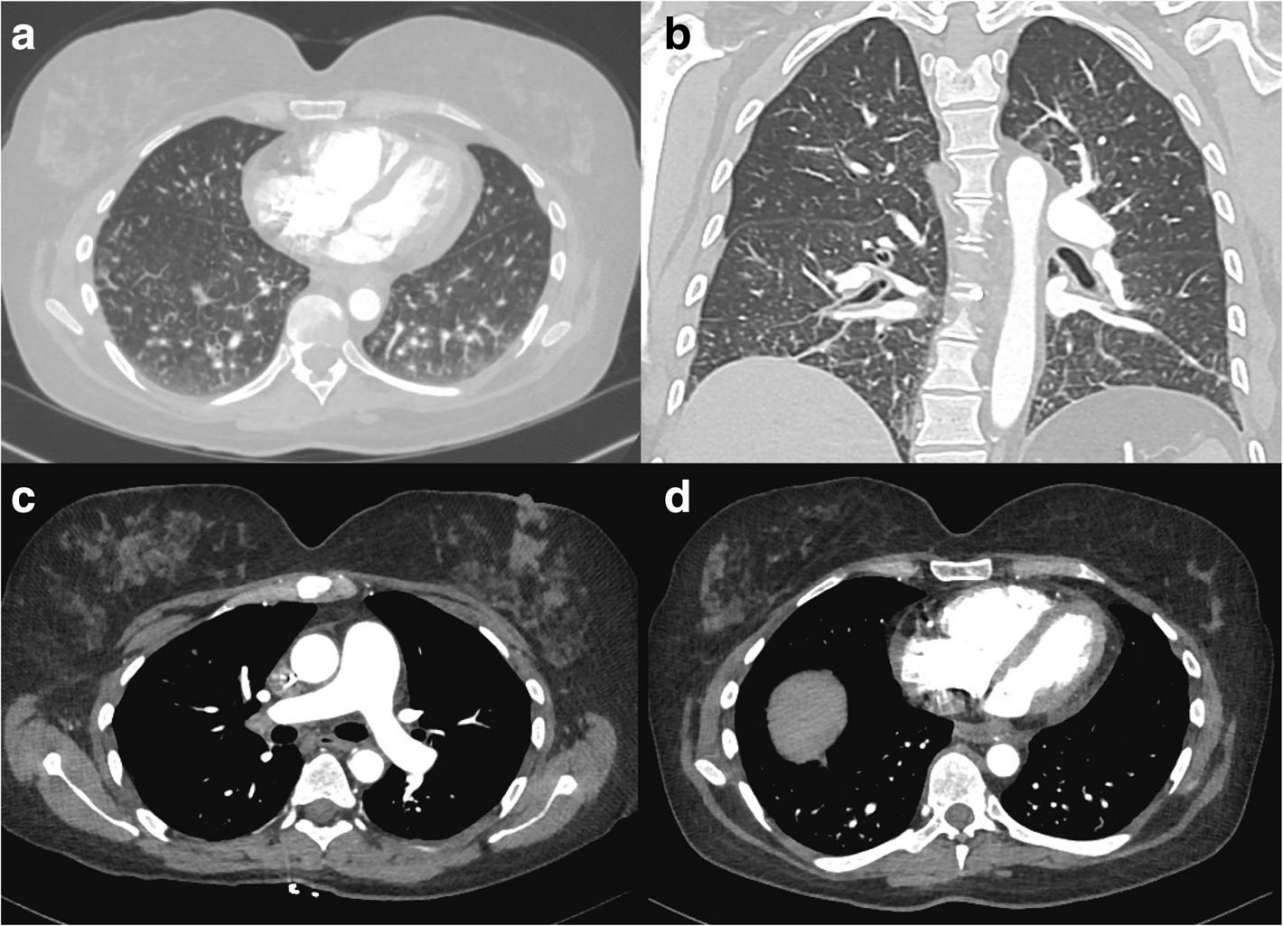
Contrast enhanced CT image with axial (a) and coronal (b) sections demonstrating a prominence of interstitial markings predominantly in posterior and basilar lung fields. In mediastinal windowing accentuating the pulmonary vasculature (c) the pulmonary artery trunk is enlarged and (d) the right ventricle and right atria appear larger in area than their corresponding left sided chambers; findings suggestive of pulmonary hypertension
